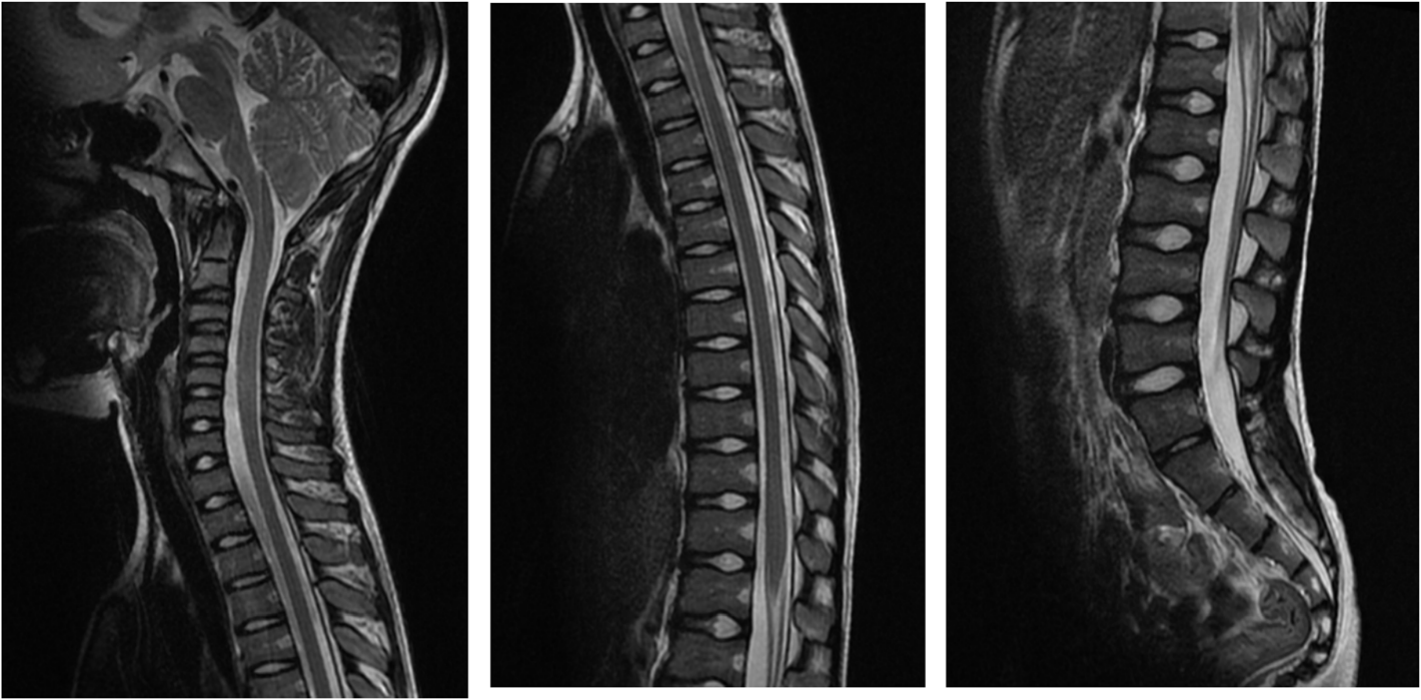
Whole-spine MRI. Whole-spine MRI revealed no abnormalities, such as Chiari malformations, syringomyelia, spinal cord cavities, spinal cord tumors, dural ectasia, and low-level conus medullaris
